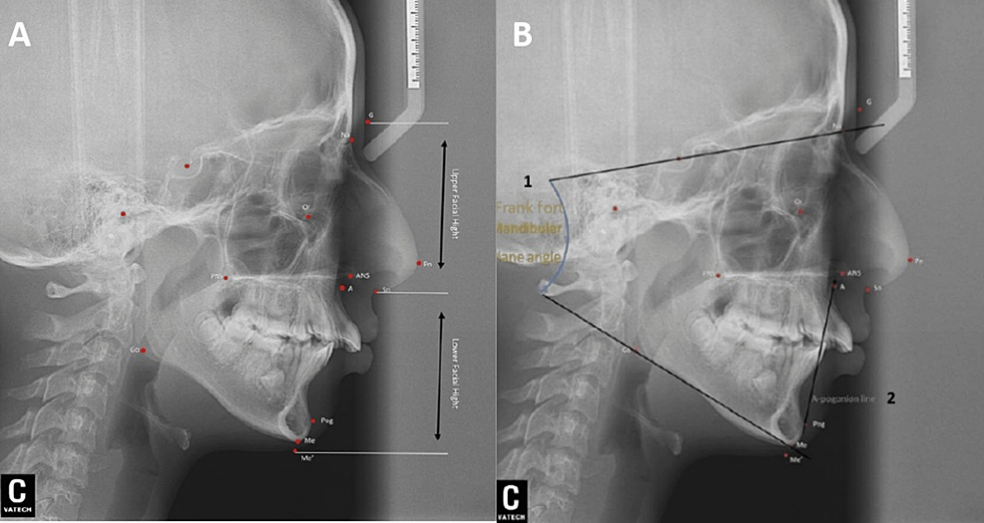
Lateral cephalometric radiographs showing the mandibular angle, pogonion line, and lower facial height (A) Lower facial height: The ratio of the distances G-Sn and Sn-Me; in normal cases, it should be approximately 1:1. (B) Frankfort-mandibular plane angle (FMA) is formed by the intersection of the Frankfort horizontal plane (FH plane) and the mandibular plane (1). Pogonion line is a line to measure the position of the anterior teeth (2). G-Sn, glabella to subnasale; Sn-Me, subnasale to menton
